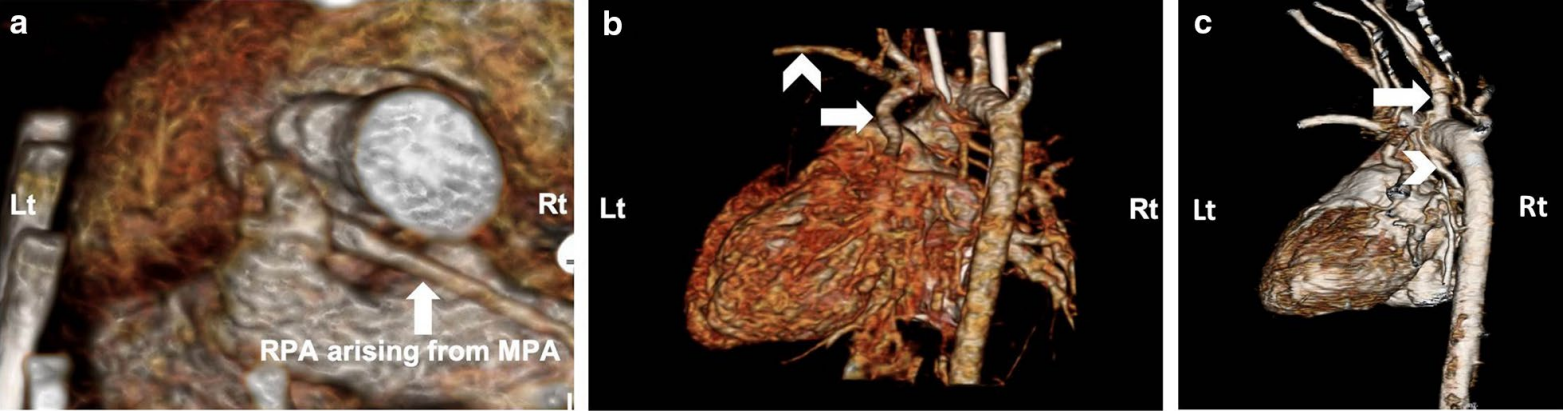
Postnatal multidetector computed tomography image showing the posterior aspect of the neonate. Also shown are the right pulmonary artery (white arrow) arising from the atretic main pulmonary artery (a) and the left pulmonary artery (white arrow) arising from the left subclavian artery (arrowhead) (b). Multiple aortopulmonary collateral arteries (MAPCAs, arrowhead) from right common carotid artery (white arrow) supply the right lung (c)
